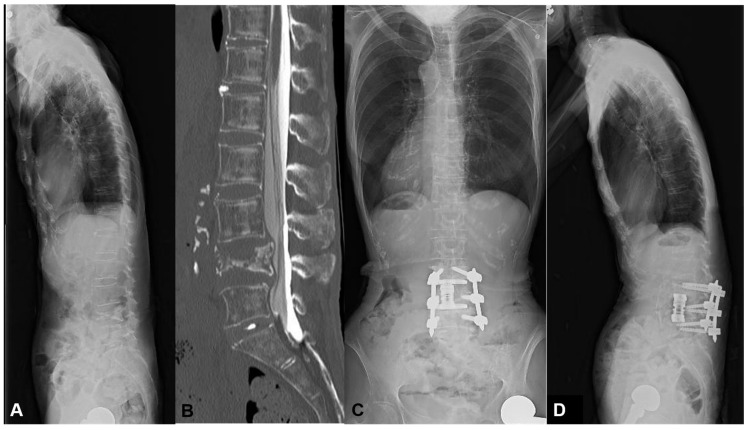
A 78-year-old female suffering from L4 vertebral collapse. The MIS L4 corpectomy followed by percutaneous screw fixation was conducted, resulting in the recovery of motor loss and gait disturbance without mechanical complications. (A) Preoperative lateral X-ray, (B) Preoperative myelogram CT, (C) Standing AP X-ray at one year postoperatively, (D) Standing lateral X-ray at one year postoperatively.
